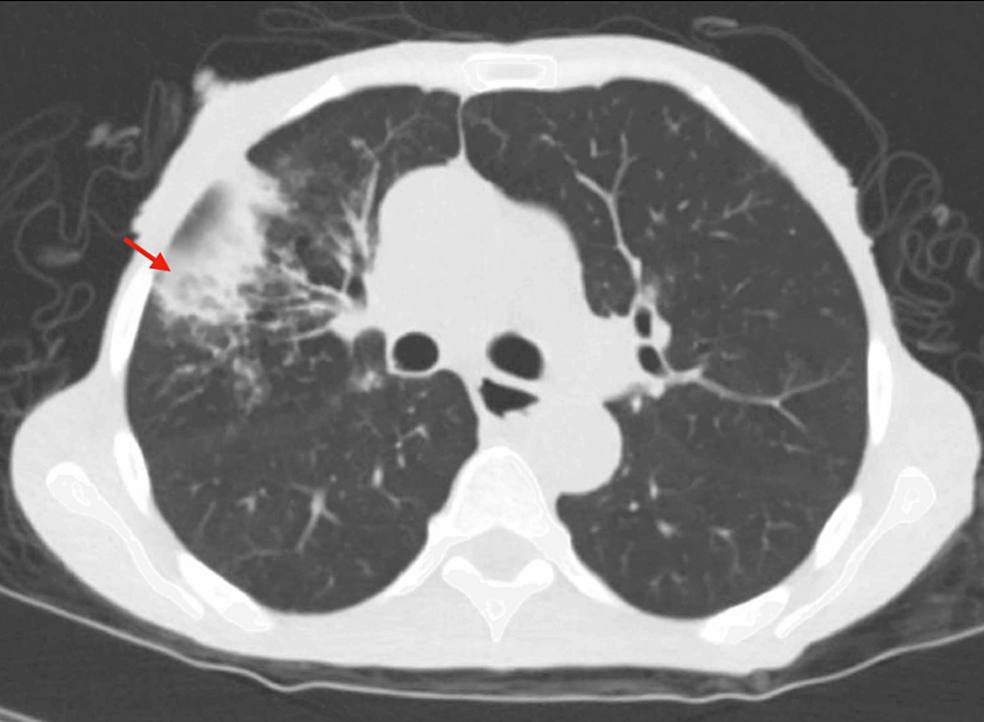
CT chest without contrast. CT was reported as interval development of branching opacities (tree-in-bud) and pleural-based areas of consolidation in the right upper and lower lobe, suggestive of pneumonia superimposed on chronic interstitial changes (as signaled by the red arrow). Additionally, these findings were also described: interval development of thickening of the minor fissure on the right. Diffuse bronchiectasis. Emphysema. Interval improvement of pleural-based opacity at the medial basal segment of the left lower lobe compared to previous imaging. Interval improvement of pleural-based opacity in the posterior basal segment of the right lower lobe compared to previous imaging.
